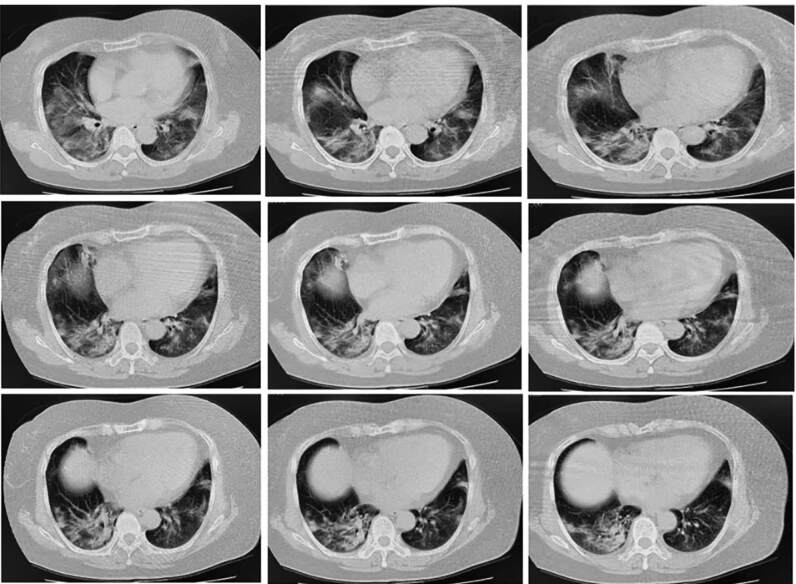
Chest CT image of the patient on February 5, 2020. Chest CT showed obvious exudative lesions in bilateral lungs.
